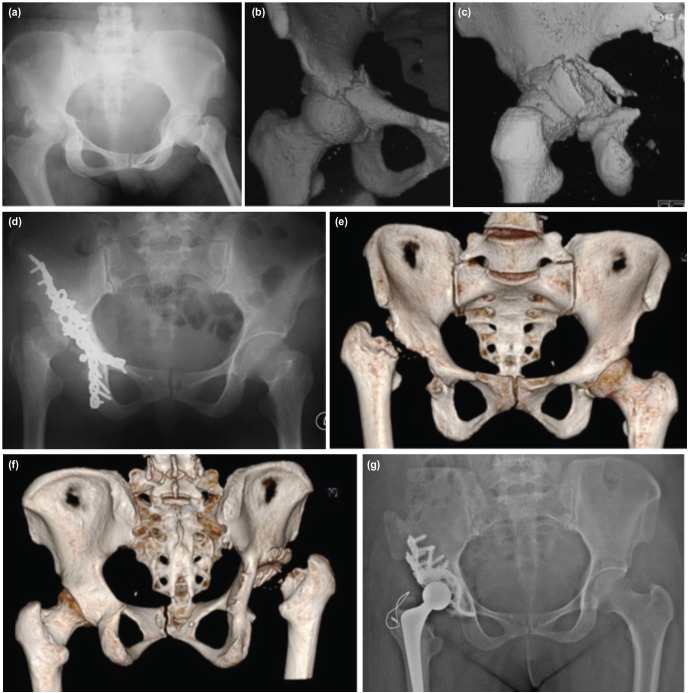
Transverse bicolumnar fracture with posterior column injury in 54-year-old lady was stabilised with double approach. It was complicated with infection requiring removal of all implants and prolonged antibiotic treatment. (a) Anteroposterior view radiograph shows a displaced transverse bicolumnar fracture with posterior column injury. (b) Closed up anteroposterior CT scan image of the fracture. The fracture line extends from the anterior column, traversing to the posterior. (c) Closed up posterior view of CT image shows comminuted medial and posterior wall. (d) Anteroposterior pelvic radiograph of bicolumnar plating of the acetabulum. This patient succumbed to implant related infection which lead to septic arthritis and AVN with femoral head lysis. (e) Anteroposterior view of CT image of right hip after removal of implant and remaining collapsed femoral head. Note part of anterior wall has undergone lysis too. (f) CT image posterior view of right hip shows the absent posterior wall of acetabulum. (g) There was no femoral head to build up the posterior wall, the illiac crest was used to build up posterior wall with impacted graft and cage cemented arthroplasty.
